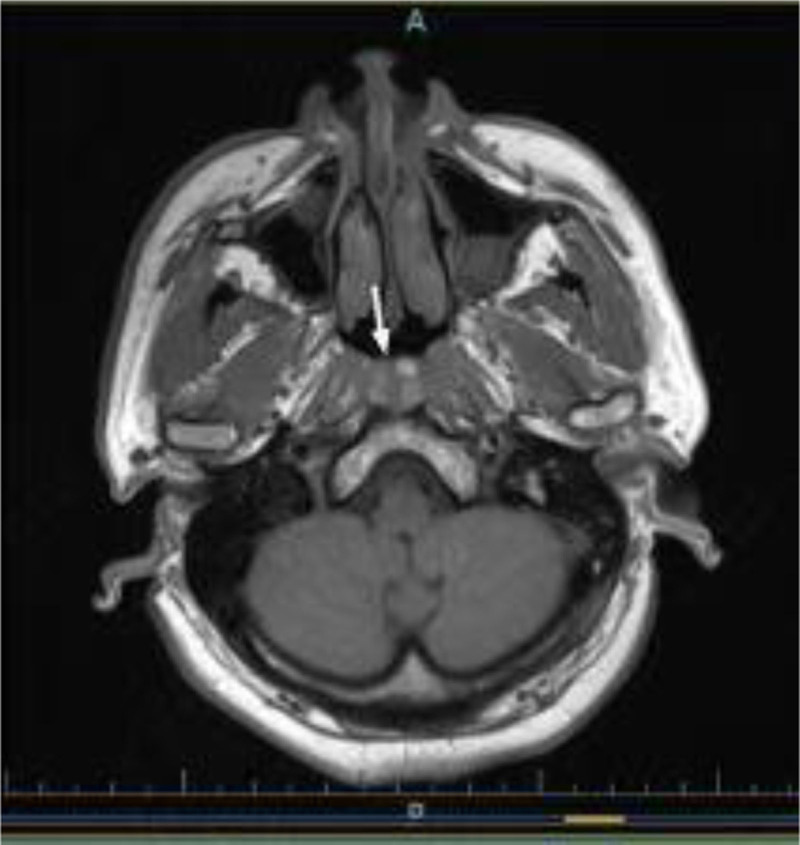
Nasopharyngeal MRI: white arrow indicate 3 nodular lesions with isointense signal. MRI = magnetic resonance imaging.
